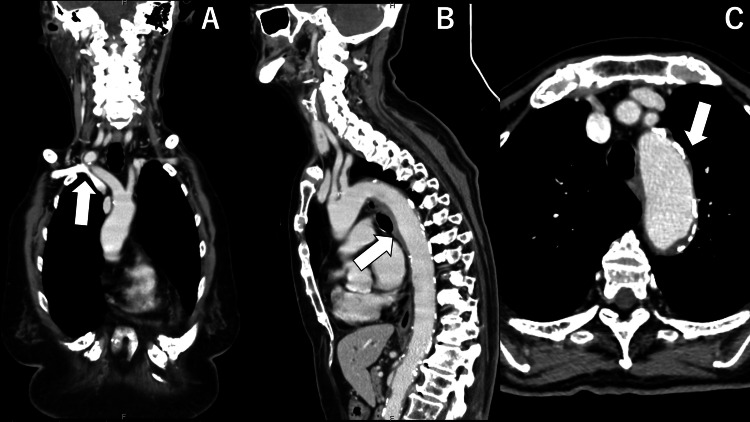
CT chest angiography, showing enhanced aortic wall from ascending to descending parts and stricture of the descending aorta and subclavian arteries (white arrows): (A) coronal, (B) sagittal, and (C) transverse views
